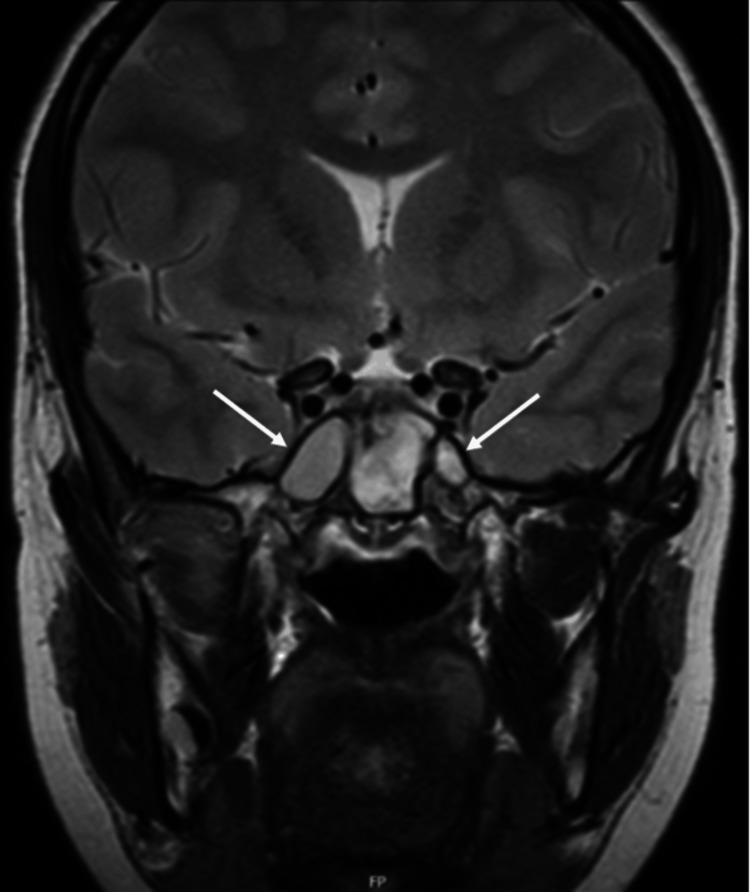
Initial coronal slice non-contrast MRI head showing extensive inflammatory changes within the sphenoid sinuses bilaterally, worse on the right
